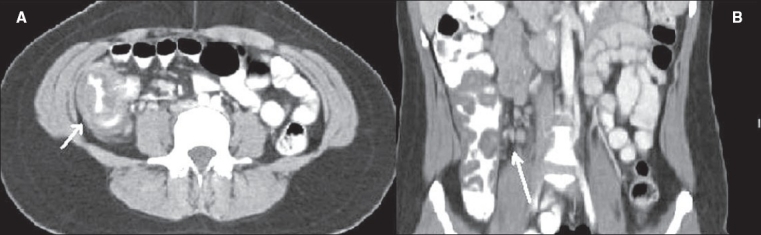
Ileocecal tuberculosis. Contrast-enhanced axial (A) and coronal (B) CT scan images show circumferential wall thickening of the cecum and terminal ileum (arrow in A) and enlarged mesenteric lymph nodes (arrow in B)
